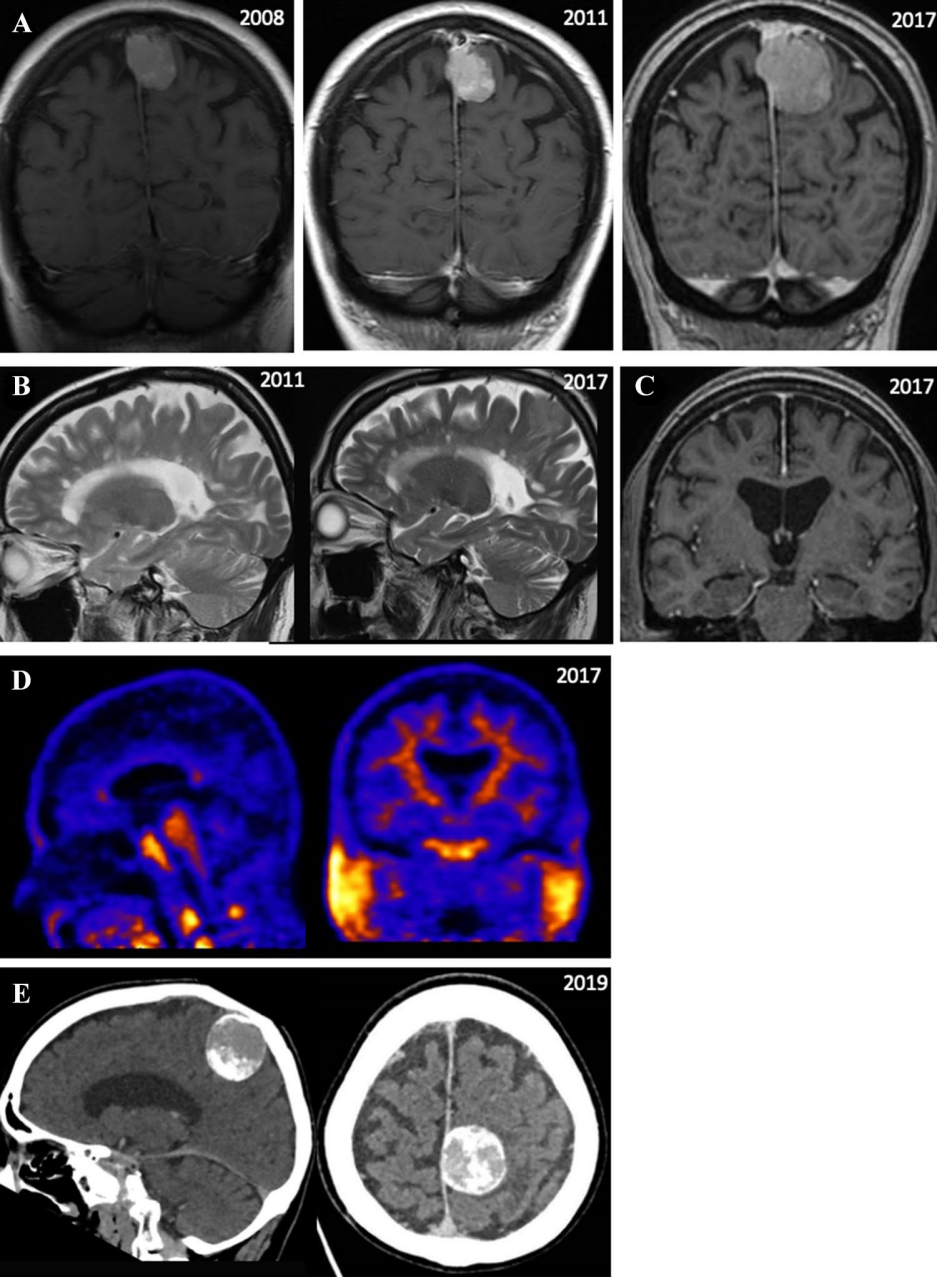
Case 2. a Post-contrast coronal T1-weighted images through the brain showing growth of the meningioma in the left parietal region. Meningioma volumes: 5.5 cc in 2008, 5.5 cc in 2011, 21 cc in 2017. b Sagittal T2-weighted MRI images demonstrating stable lesion load in the periventricular white matter between 2011 and 2017. c Coronal post-contrast T1-weighted MRI image demonstrating normal bilateral hippocampal volumes at age 76. d Negative amyloid PET scan, with clear differentiation between grey and white matters and absence of tracer uptake in the cerebral grey matter. e CT scan showing an interval increase in size of the left parasagittal meningioma (4.3 × 3.7 × 3.5 cm) with associated local mass effect and vasogenic oedema
